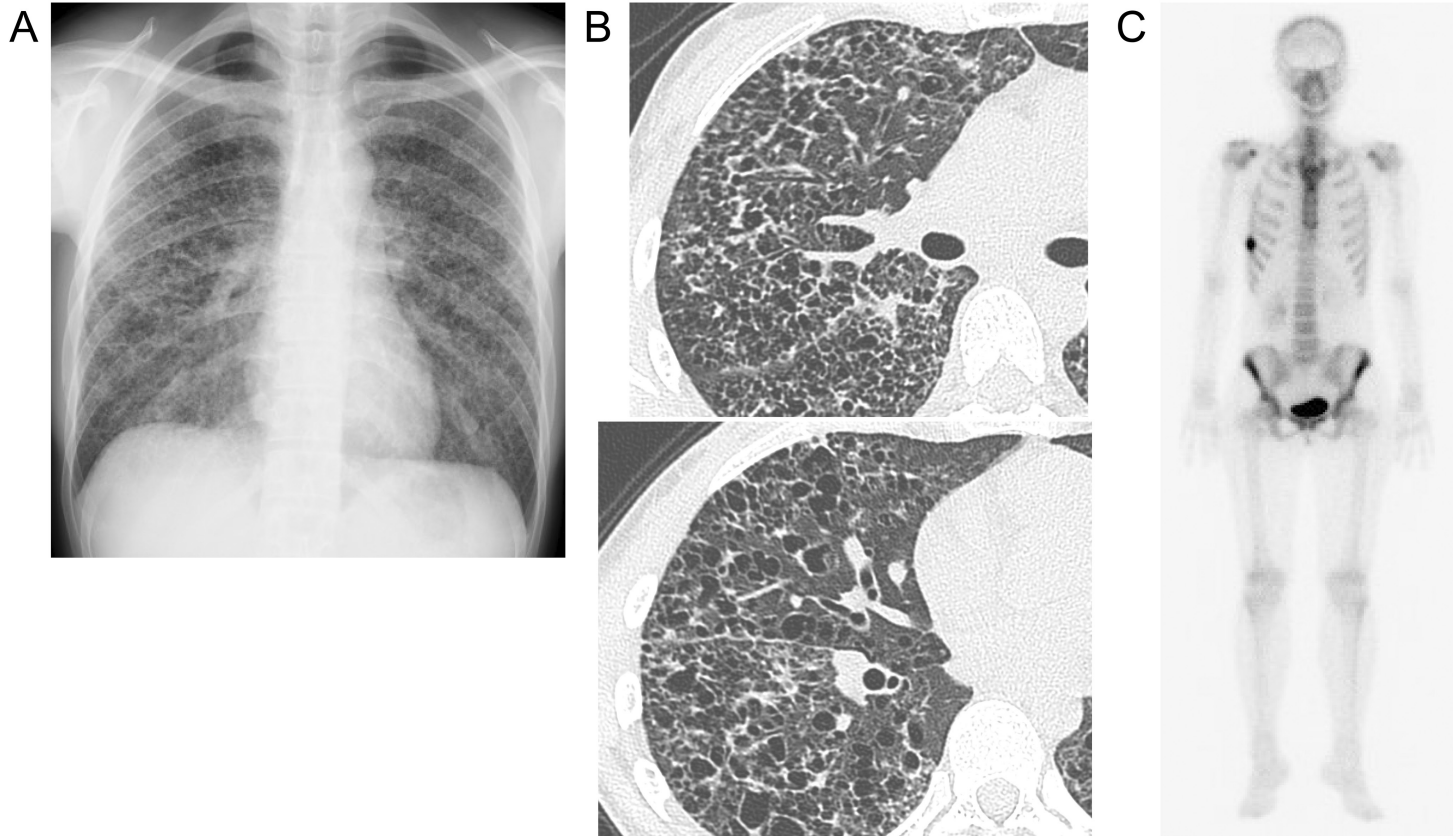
Chest radiograph showing bilateral diffuse ground‐glass opacities (A) Chest CT showing multiple bilateral cystic lesions with granular shadows (B) Brain MRI showed no intracranial lesions and mass‐like uptake in the right 10th rib on bone scintigraphy (C).
